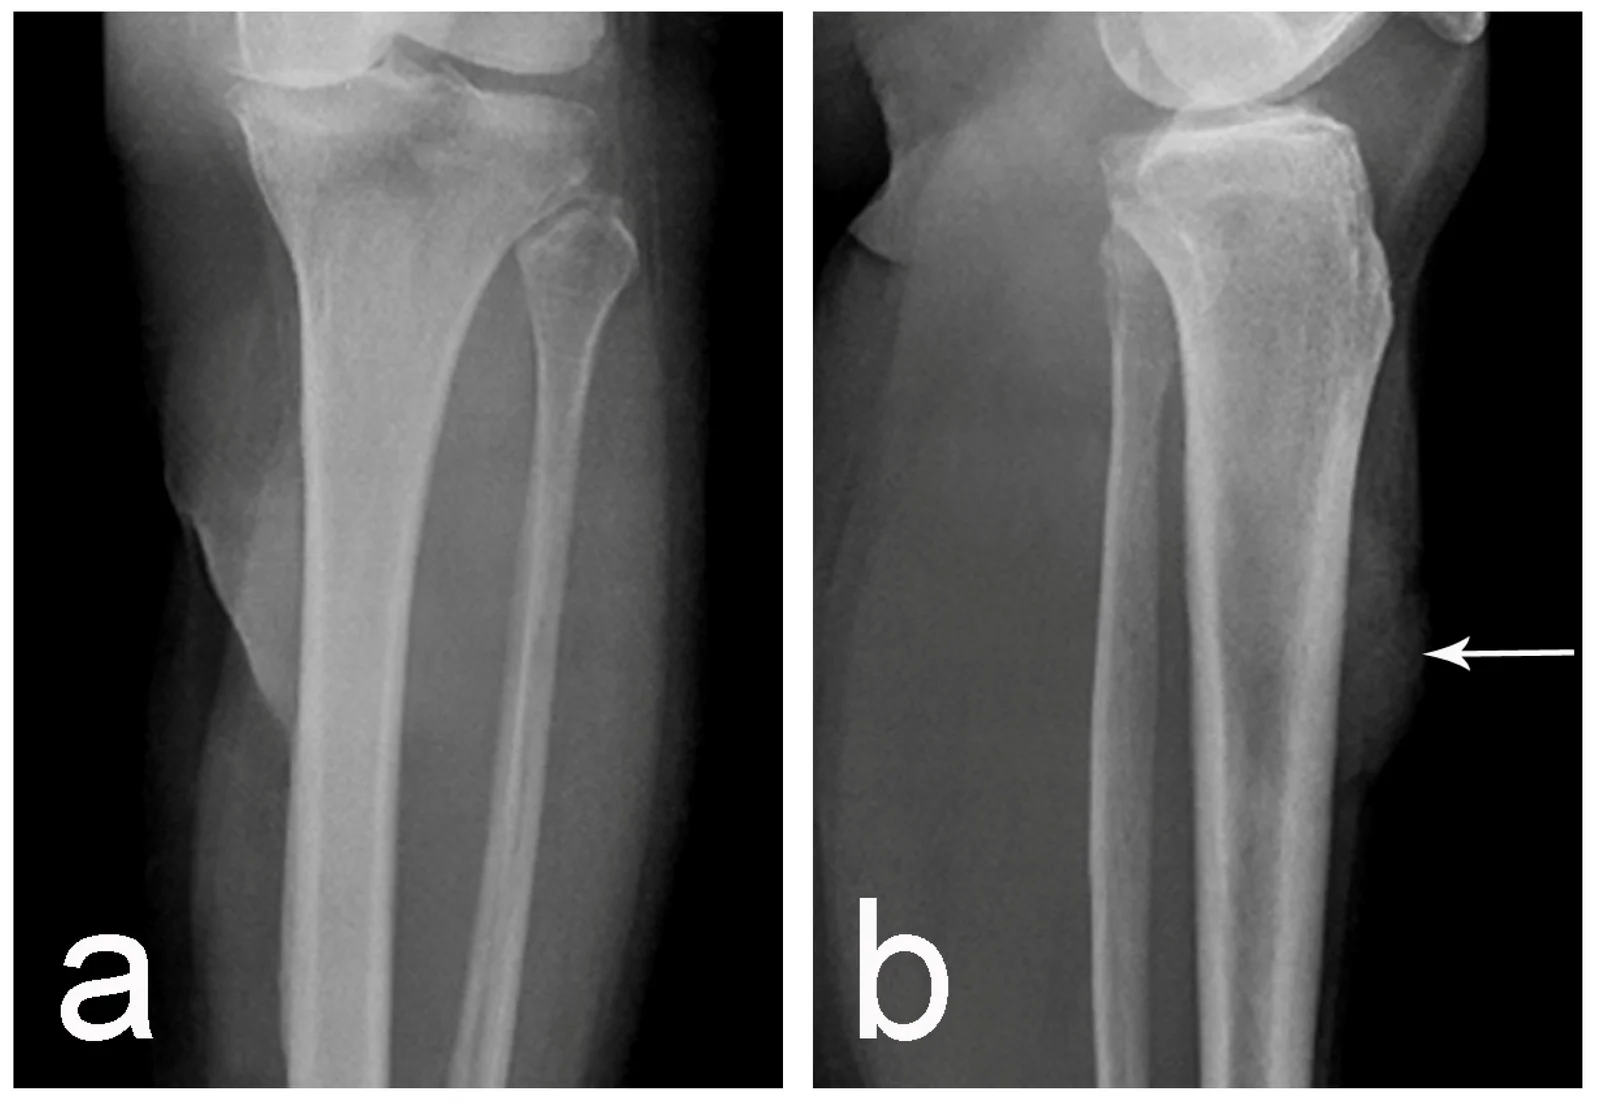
Preoperative anteroposterior (a) and lateral (b) radiographs of the proximal tibia demonstrating the baseline osseous condition before definitive resection as well as the soft-tissue component (arrow).
